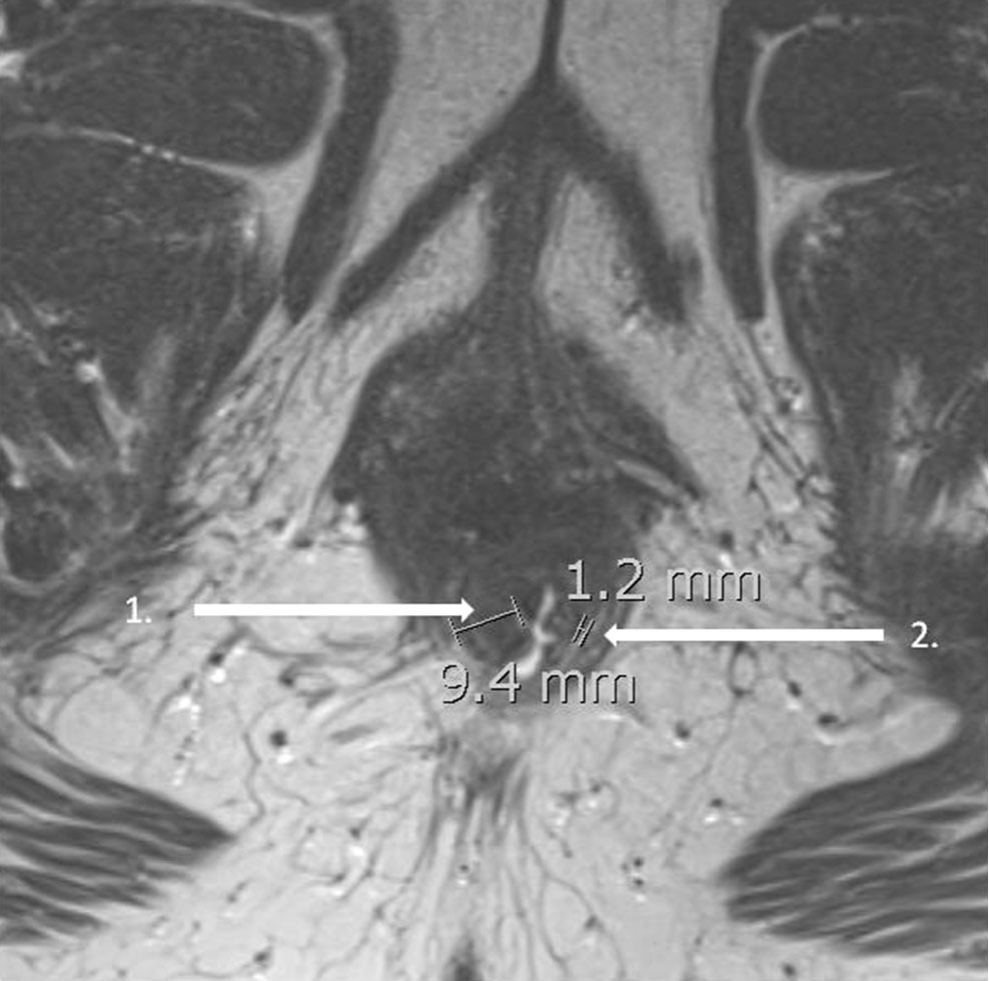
Axial T2-weighted turbo spin-echo MRI showing anal sphincter measurements in a 49-year-old woman.* 1* total sphincter thickness (9.4 mm);* 2* hyperintense intersphincteric fat (1.2 mm)
